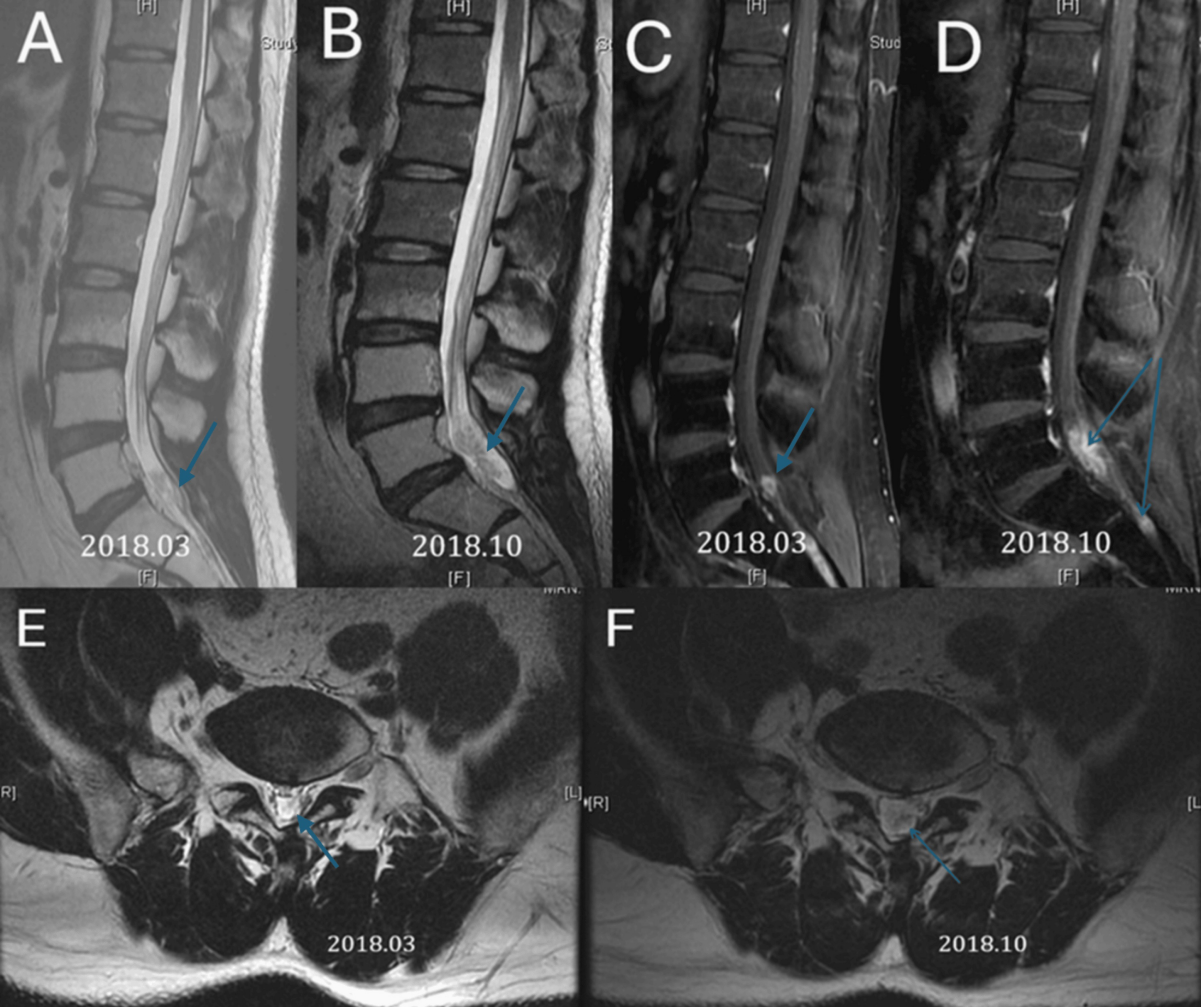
(A) T2-weighted MRI: An intradural hyperintense lesion is observed in the cauda equina region, causing mild compression of the nerve roots. At this stage, the patient experienced left-sided sciatica. (B) T2-weighted MRI reveals further enlargement of the lesion, with increased compression of the cauda equina nerve roots and more pronounced spinal canal narrowing. The patient's symptoms worsened, with progressive left foot drop and increased pain, prompting the decision for surgical intervention. (C-D) Contrast-enhanced T1-weighted MRI. (E-F) Axial T2-weighted MRI showing an intradural lesion compressing the cauda equina nerve roots.
